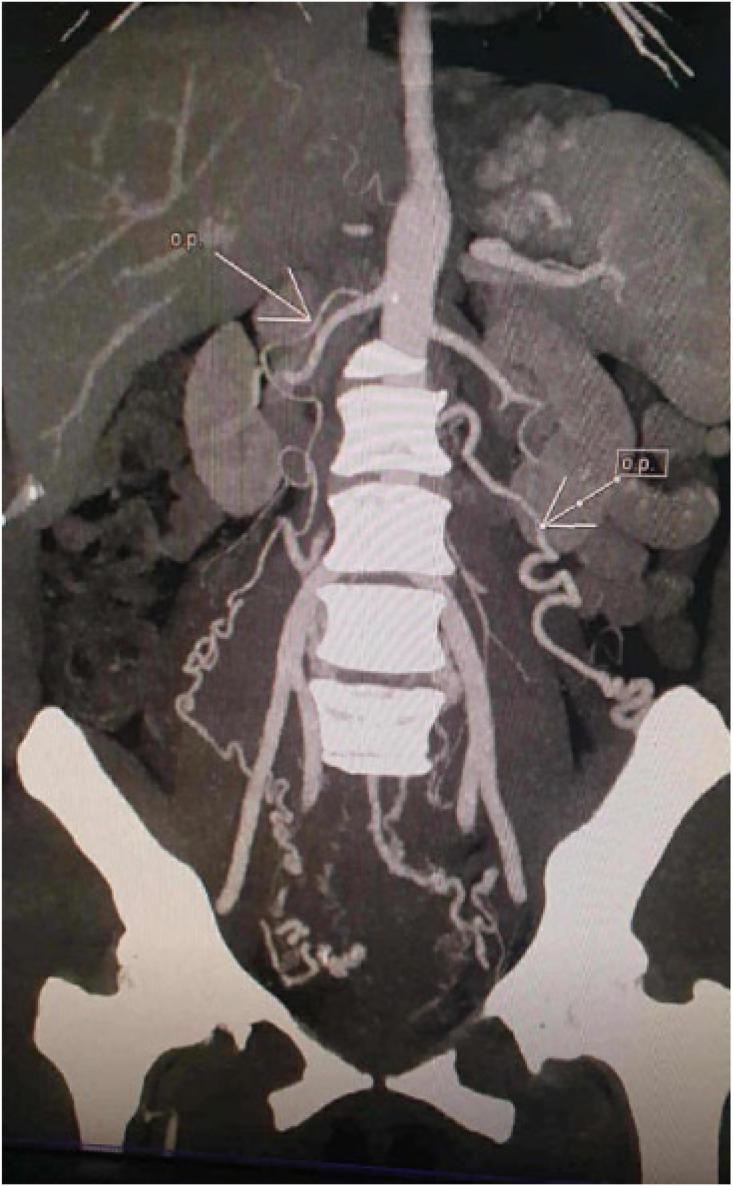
Contrast-enhanced CT image coronal image demonstrate a large heterogeneous mass with a pelvic venous reflux involving the left ovarian vein and the right internal iliac vein (Arrows).
